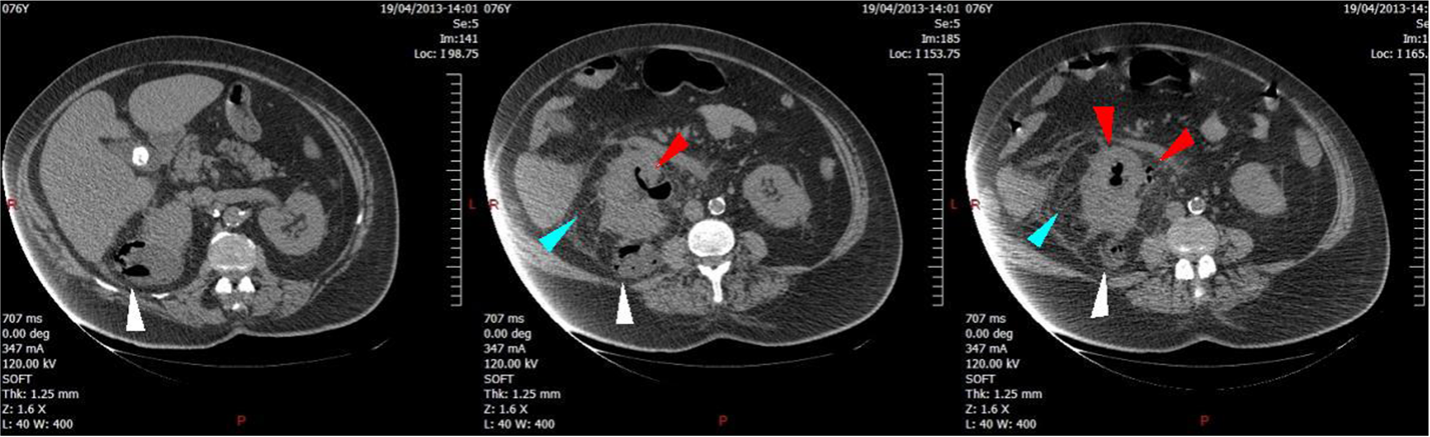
Emphysematous pyelonephritis (EPN) of the right kidney. Computed tomography scan demonstrates right-sided EPN with enlarged right kidney and normal left kidney. Gas is present in the renal pelvis, in the proximal ureter (red arrows), and in a posterior cyst of the right kidney (white arrows). There is also significant perirenal infiltration (blue arrows).
